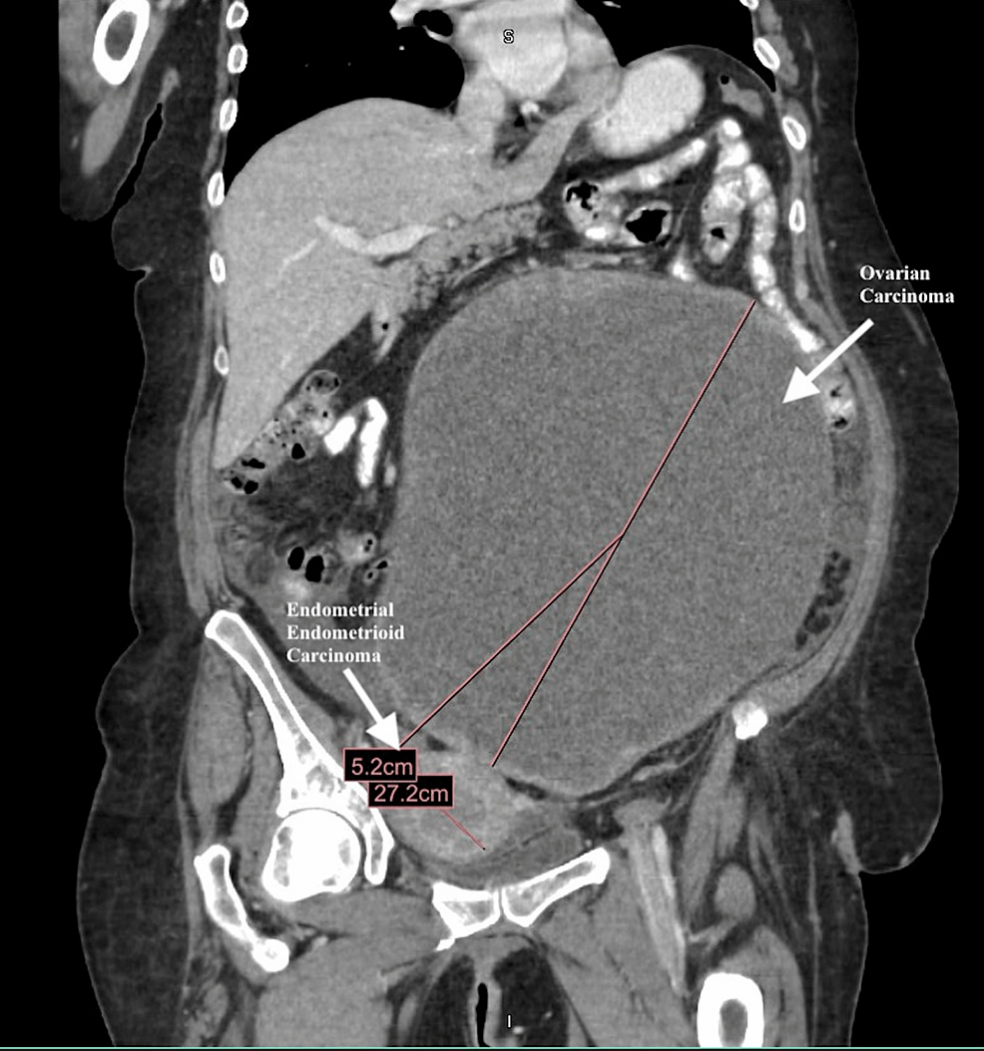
Abdominal CT scan presenting the 27 cm diameter cystic mass in greatest dimension (arrow). Her uterus is also prominent with a diameter of 5.2 cm (arrow). CT: computed tomography
